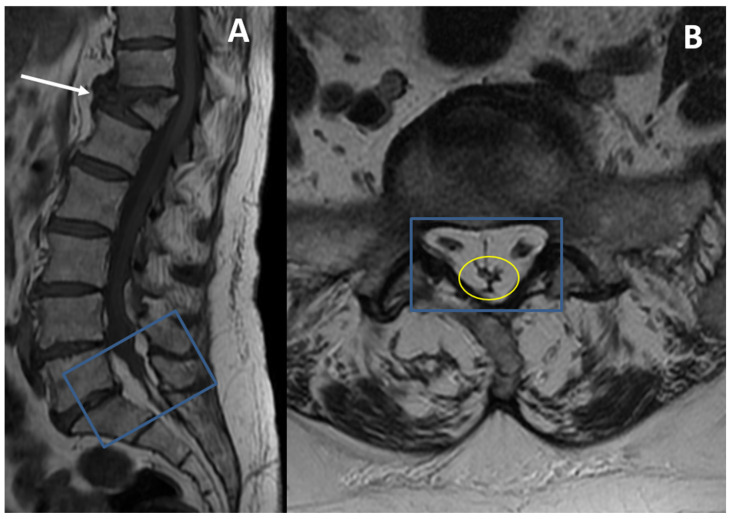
A 72-year-old woman affected by dyslipidemia, obesity, and high blood pressure underwent follow-up MRI control for a vertebral fracture (T12); patient complained about chronic lumbar pain, which worsened after the fracture, and bilateral sciatica. (A) Sagittal T1-weighted imaging (WI); (B) axial T1-WI. A marked vertebral body collapse of the T12 vertebra is detectable (white arrow). Moreover, a condition of SEL at L5-S1 level (blue rectangles) with compression of the thecal sac resembling the letter “Y” (yellow oval) is found.
